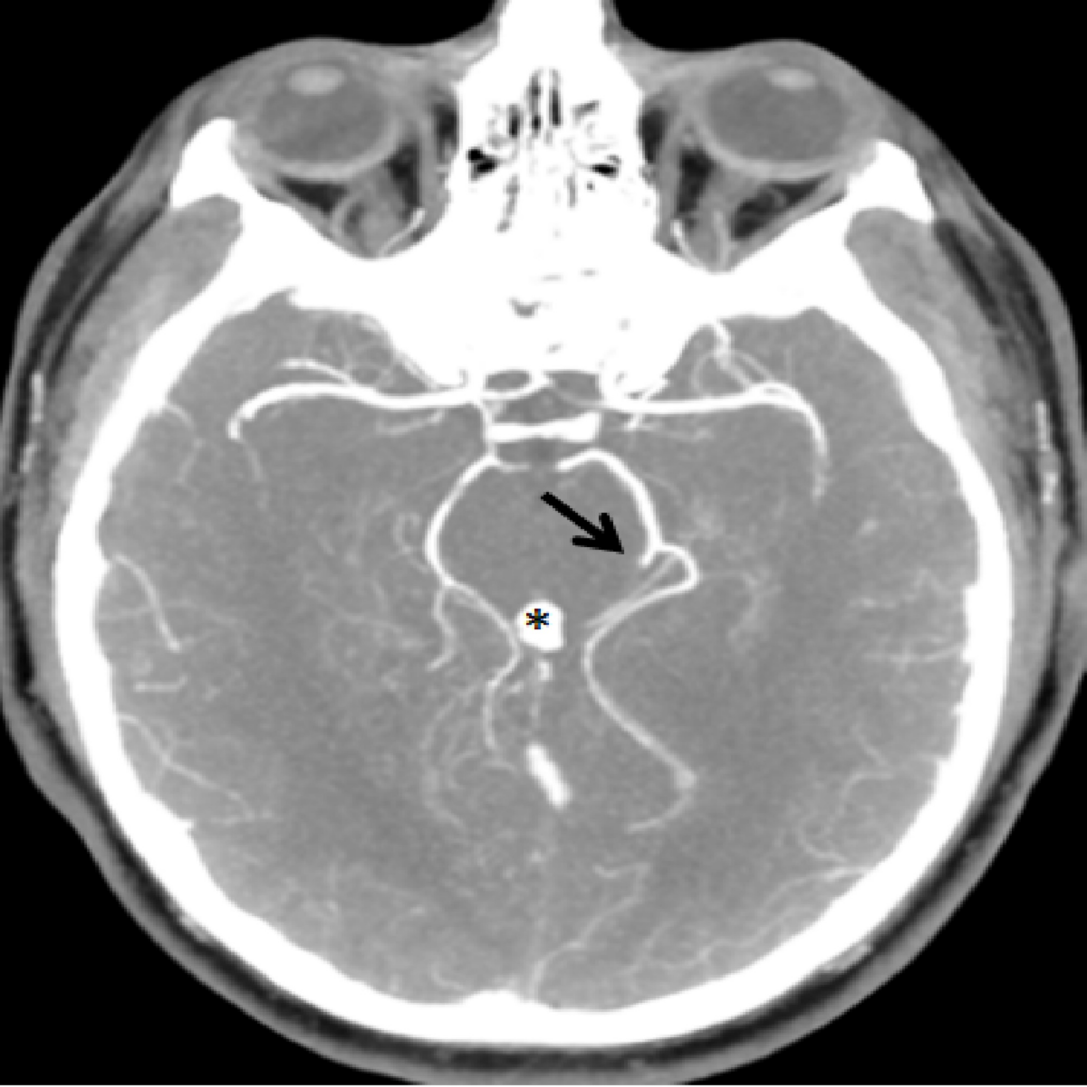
Maximum intensity projection of CT arteriography showing occlusion of a branch of the left posterior cerebral artery (arrow).
